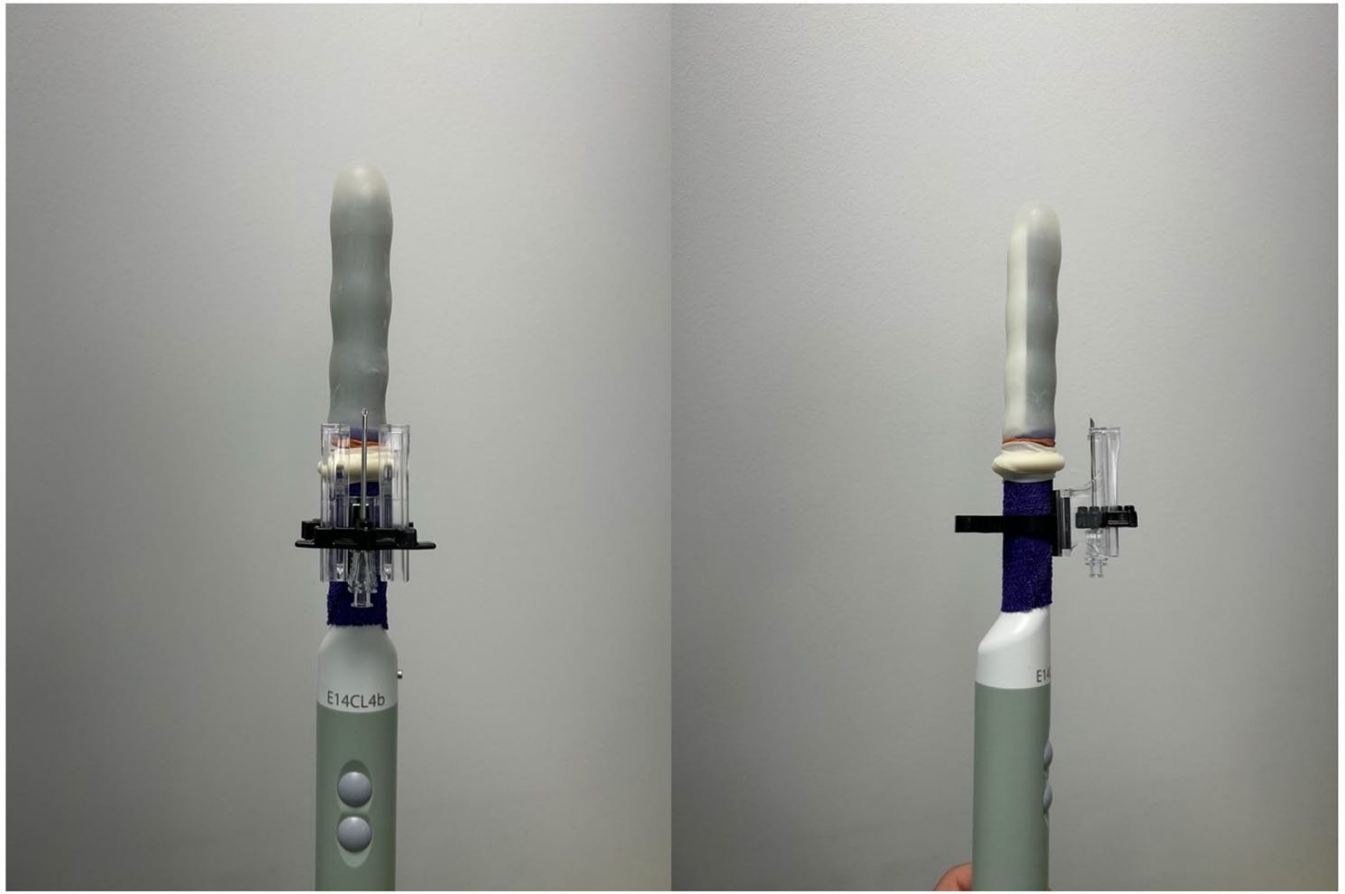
Biplane linear ultrasound probe 7 Hz with 3 M™ Coban™ wrapped around proximal shaft and a transducer cover with ultrasound gel secured with rubber bands at the base. PrecisionPoint access system over proximal shaft
